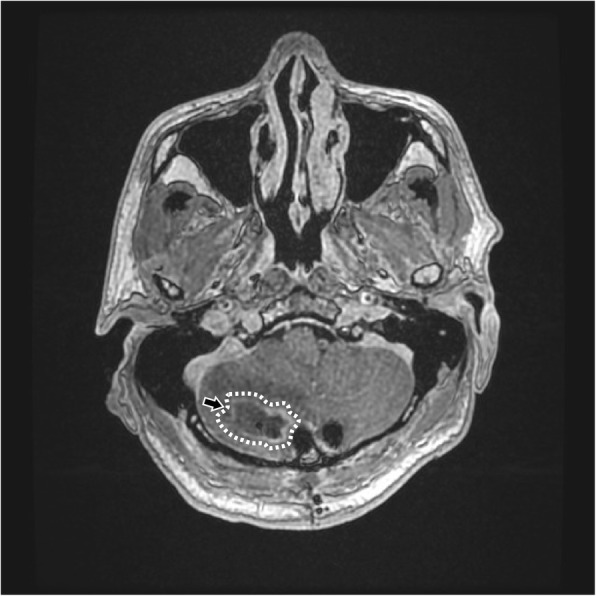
The appearance of cerebellar abscess at MRI image. MRI confirmed the diagnosis of a 2.5 cm of a faint ring-enhanced cerebellar abscess based on T1-weighted images with contrast medium. Circled area was represented as brain abscesses
